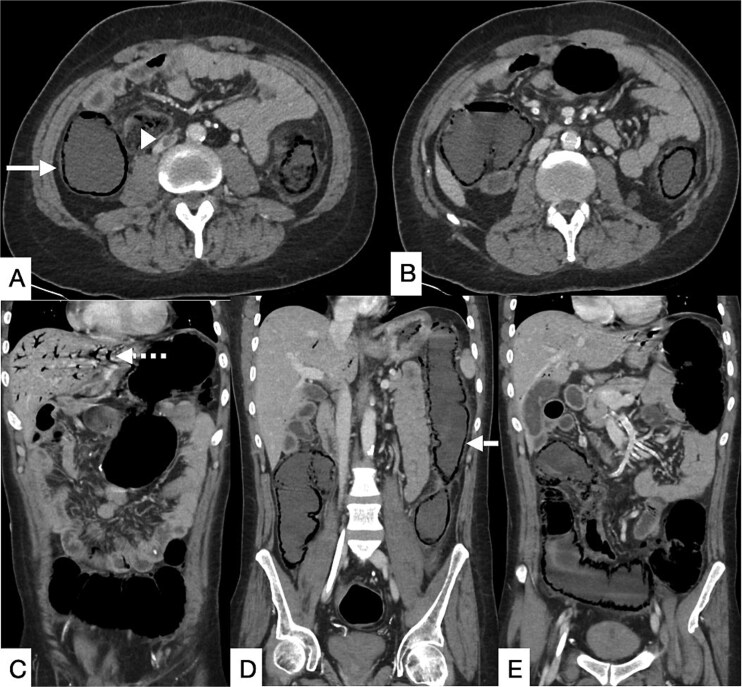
Contrast-enhanced CT of the abdomen and pelvis in axial (A, B) and coronal (C–E) planes showing a long segment of pneumatosis intestinalis involving the remaining descending colon, transverse colon, and proximal sigmoid colon, with suspicion of bowel perforation (bold arrow). Pneumobilia is present (dashed arrow). An eccentric intraluminal hypodensity within the IVC (arrowhead) is consistent with a non-occlusive thrombus, with a possible thrombus in the inferior mesenteric vein.
